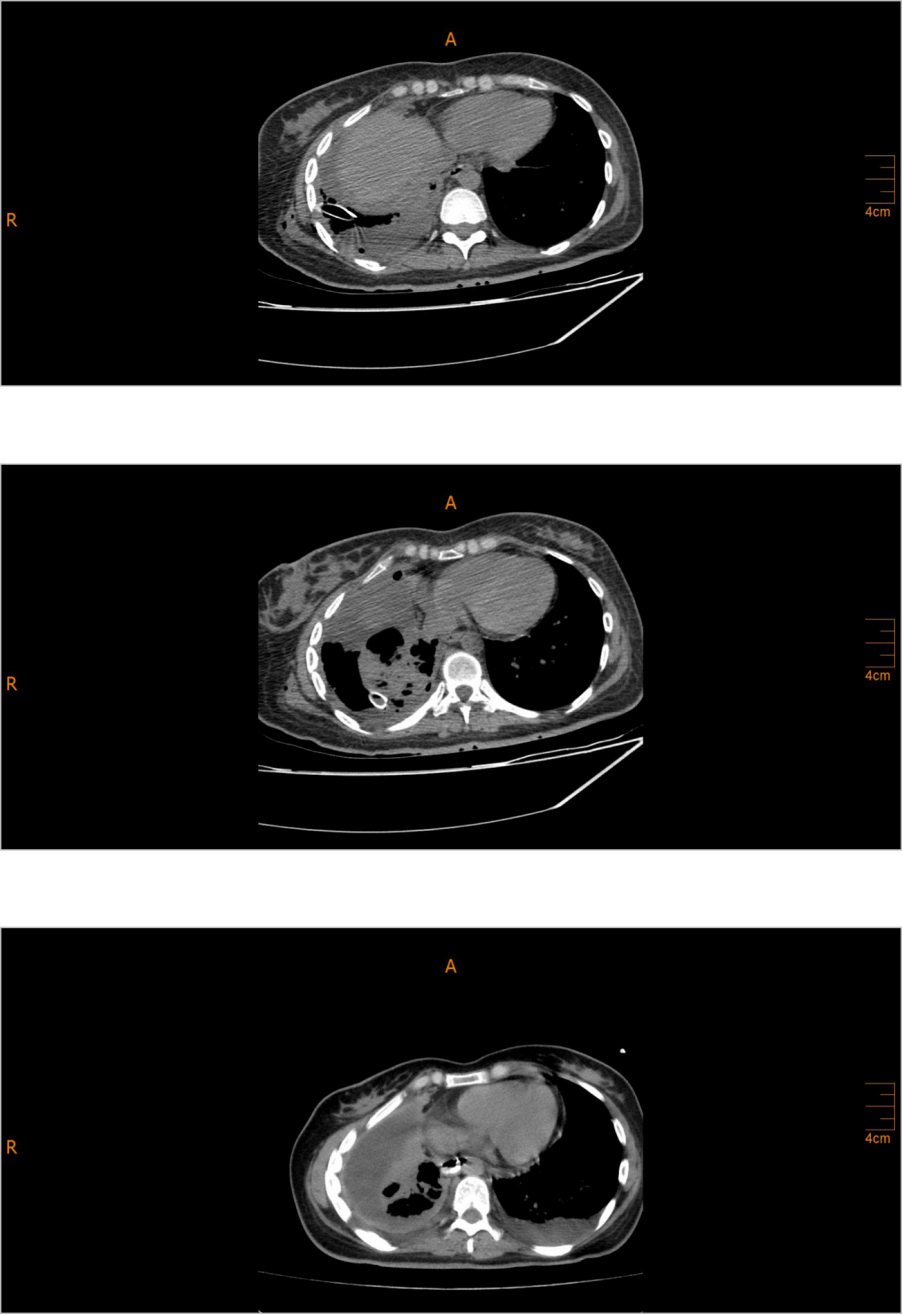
2022/08/10—spiral lung CT showing pleural effusion and a right perihilar consolidation causing the collapse of the RLL in the right hemithorax.
